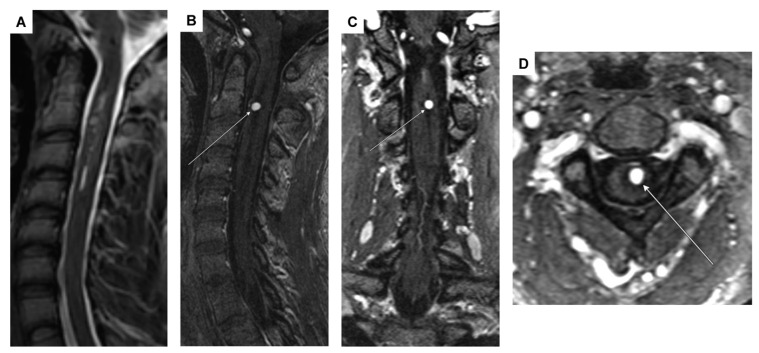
MRI showing a 4 mm intramedullary arterial aneurysm (arrows) with homogeneous contrast enhancement at the C2 vertebral base level and associated spinal cord edema/malacia from C2 to C4, likely post-hemorrhagic in origin. (A) Sagittal T_2_-weighted sequence; (B–D) arterial-phase contrast-enhanced MR angiography in the sagittal, coronal, and transversal planes.
